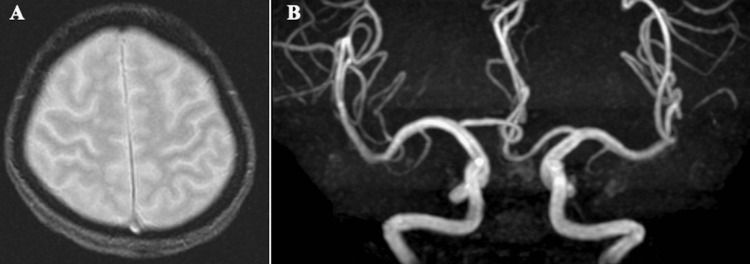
A. Magnetic resonance imaging of the head was performed 13 years prior to the patient's current presentation demonstrated no cerebral microbleeds on T2-weighted imaging; B. Magnetic resonance angiography of the anterior circulation showed no abnormal vessels or aneurysms.
